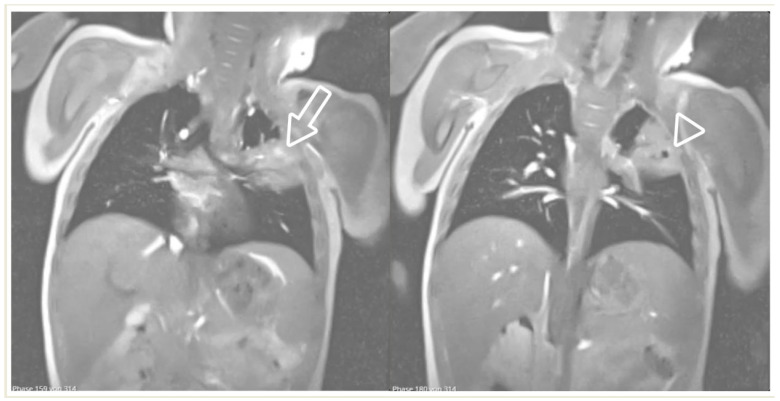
Real-time MRI sequence in the coronal plane in a 1-year-old girl with left upper-lobe pneumonia. Selected images from the free-breathing scan, which took 16 s to acquire and reconstruct (Supplementary Materials section). Within the isointense pulmonary infiltrate, there are areas of bright signal intensity (arrow) and a round area of very low (almost black) signal intensity, suggestive of air inclusions (arrowhead), with an appearance consistent with cavitary necrosis.
